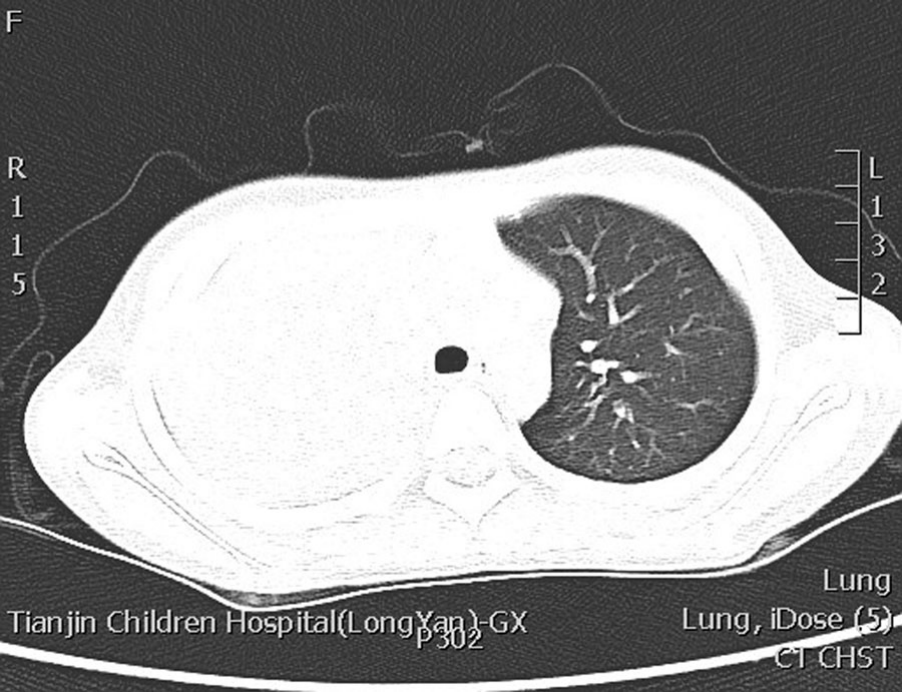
Chest computed tomography of patients showed large consolidated, accompanied with pulmonary atelectasis.
